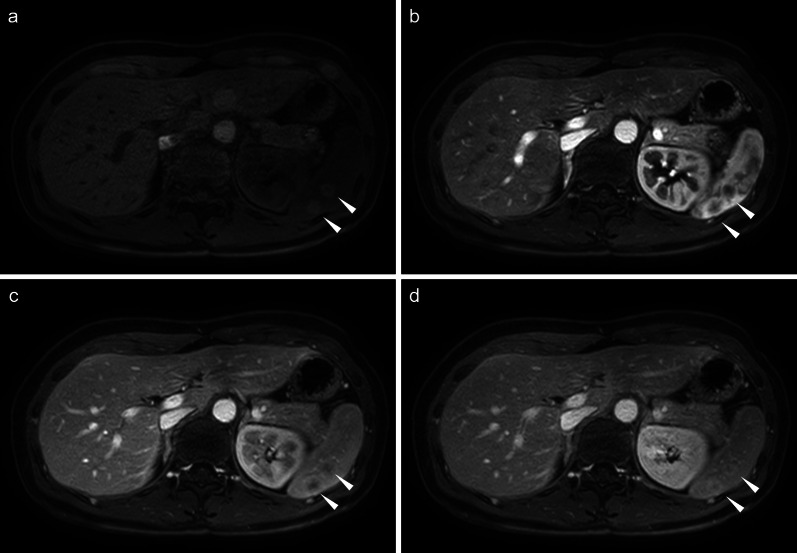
Magnetic resonance imaging findings of case 2. a Multiple ring-shaped, high-signal nodules are observed in the spleen on T1-weighted images. b Early phase, c late phase, and d delayed phase of the dynamic study. Arrowheads indicate splenic tumors
